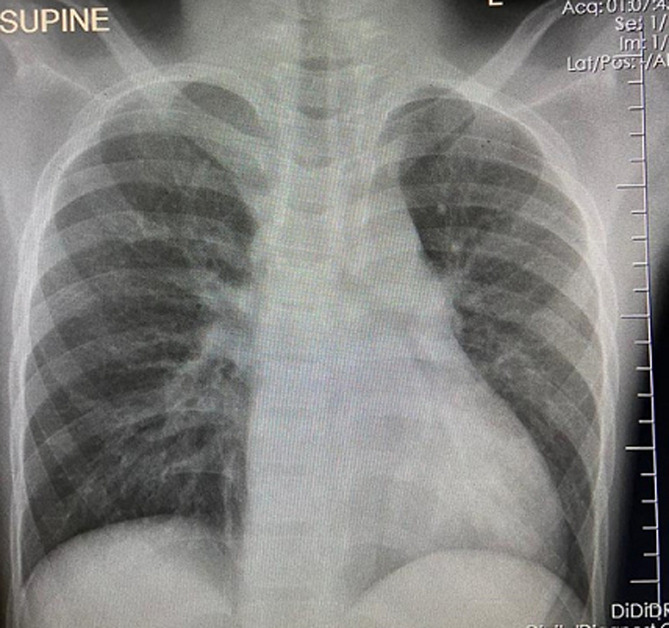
chest radiograph of the patient
